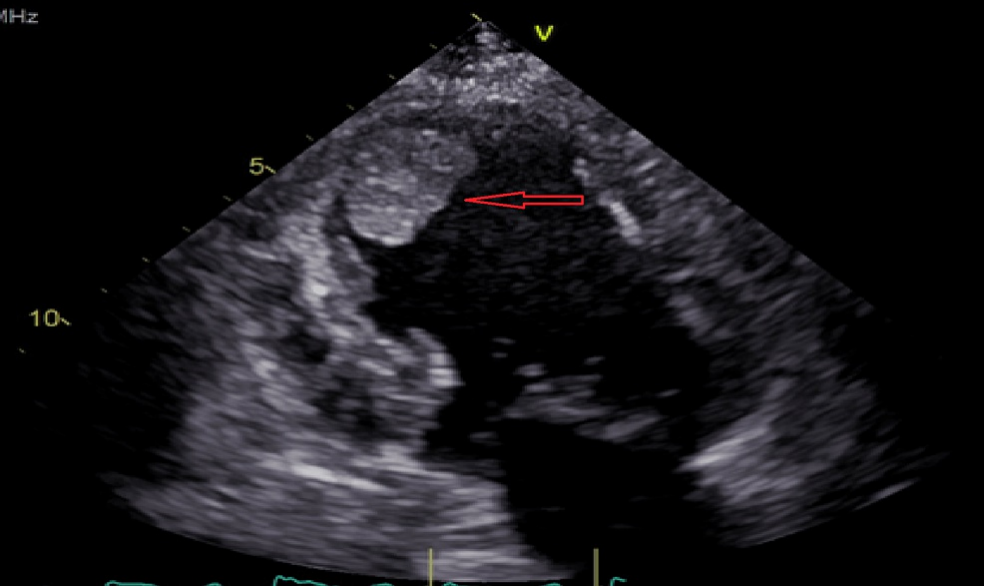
Echocardiography showing thrombus in the left ventricle.
